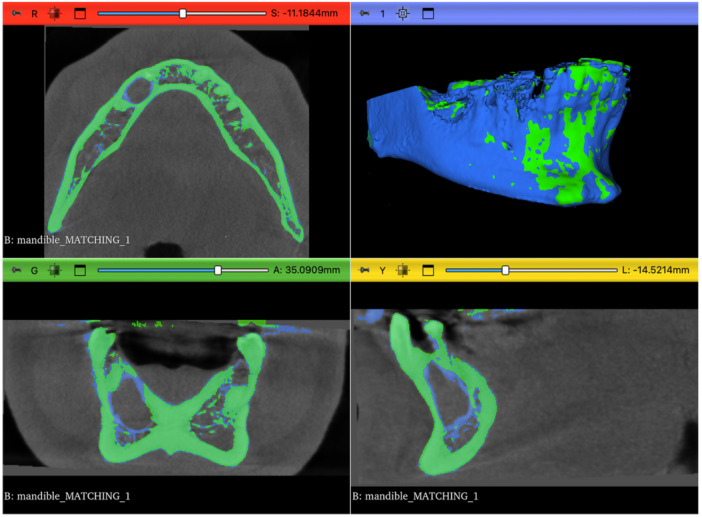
Visual assessment of CBCT co‐registration between baseline (T0, green) and follow‐up (T1, blue) mandibular volumes in axial, coronal, and sagittal planes.
